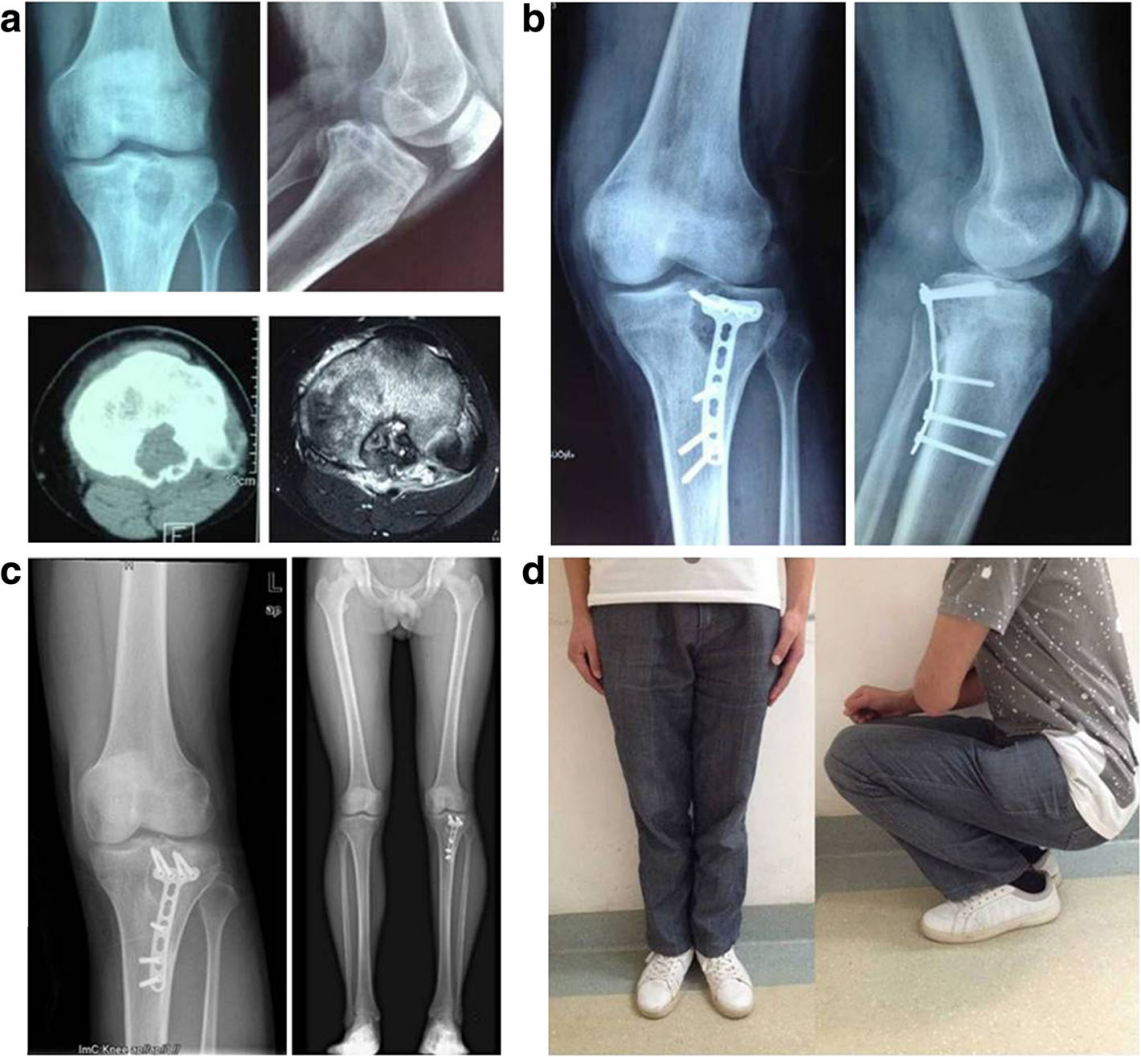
A 15-year-old boy’s X-ray , CT , MRI (a)showed the lesion located at posterior median of the proximal tibia. X-ray at 1 year(b) and 9.5 years(c) postoperative told us that the grafting bone had been gradually incorporated with the host bone and that the troubled limb shortened 20 mm compared with the healthy limb(d). The patient acquired a satisfactory function outcome and could run 3 min 43 s in 1-km race
